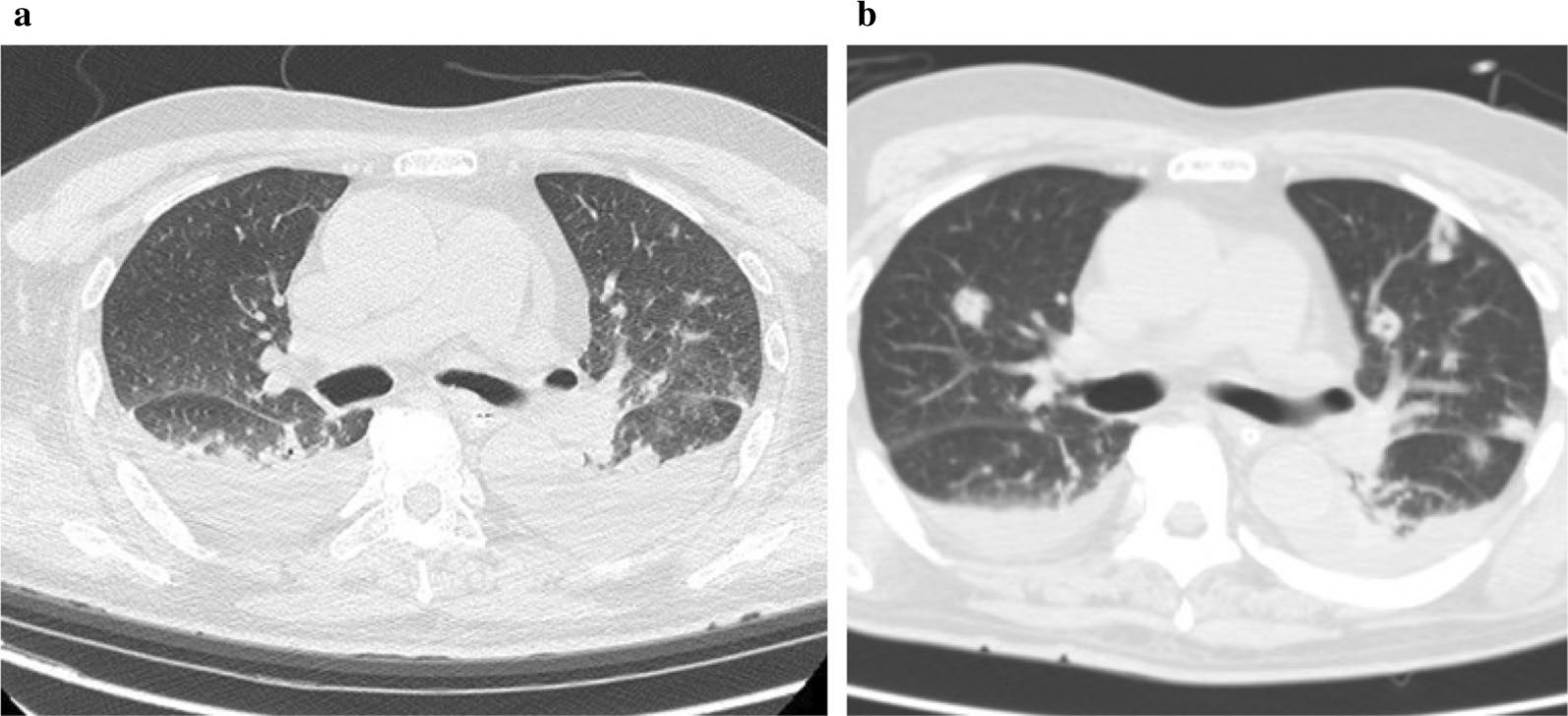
a Computed tomography scan performed 9 days before living-donor liver transplantation. Only a small amount of pleural effusion was present. b Computed tomography scan performed 3 days before living-donor liver transplantation. Some small nodular shadows were observed
